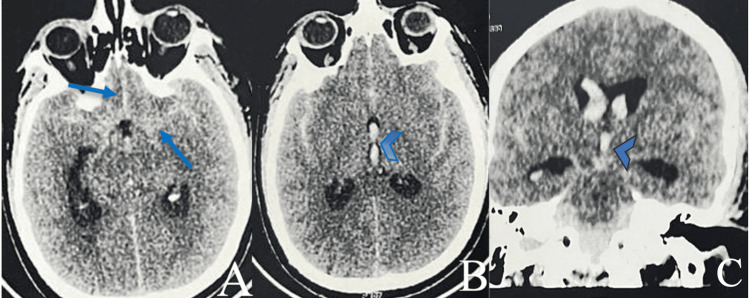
Brain CT scan: axial plane (A, B) without contrast and coronal plane (C) post-contrast injection. (A) Diffuse subarachnoid hemorrhage of the basal cistern (arrow) with (B, C) ventricular flooding into the third ventricle (arrowhead) (grade 4 on the modified Fisher scale).
